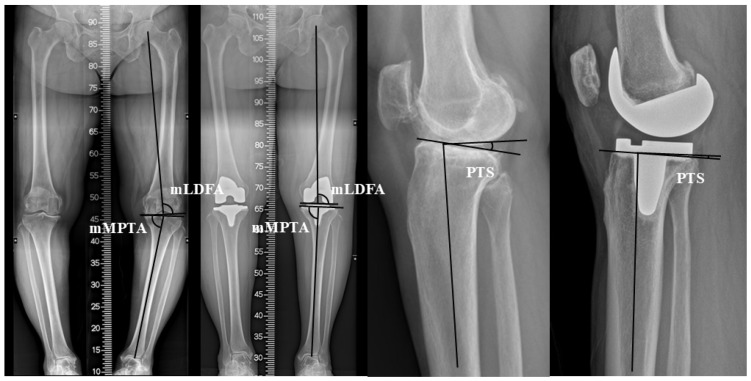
Radiographic measurements. mLDFA, mechanical lateral distal femoral angle; mMPTA, mechanical medial proximal tibial angle; PTS, posterior tibial slope.
